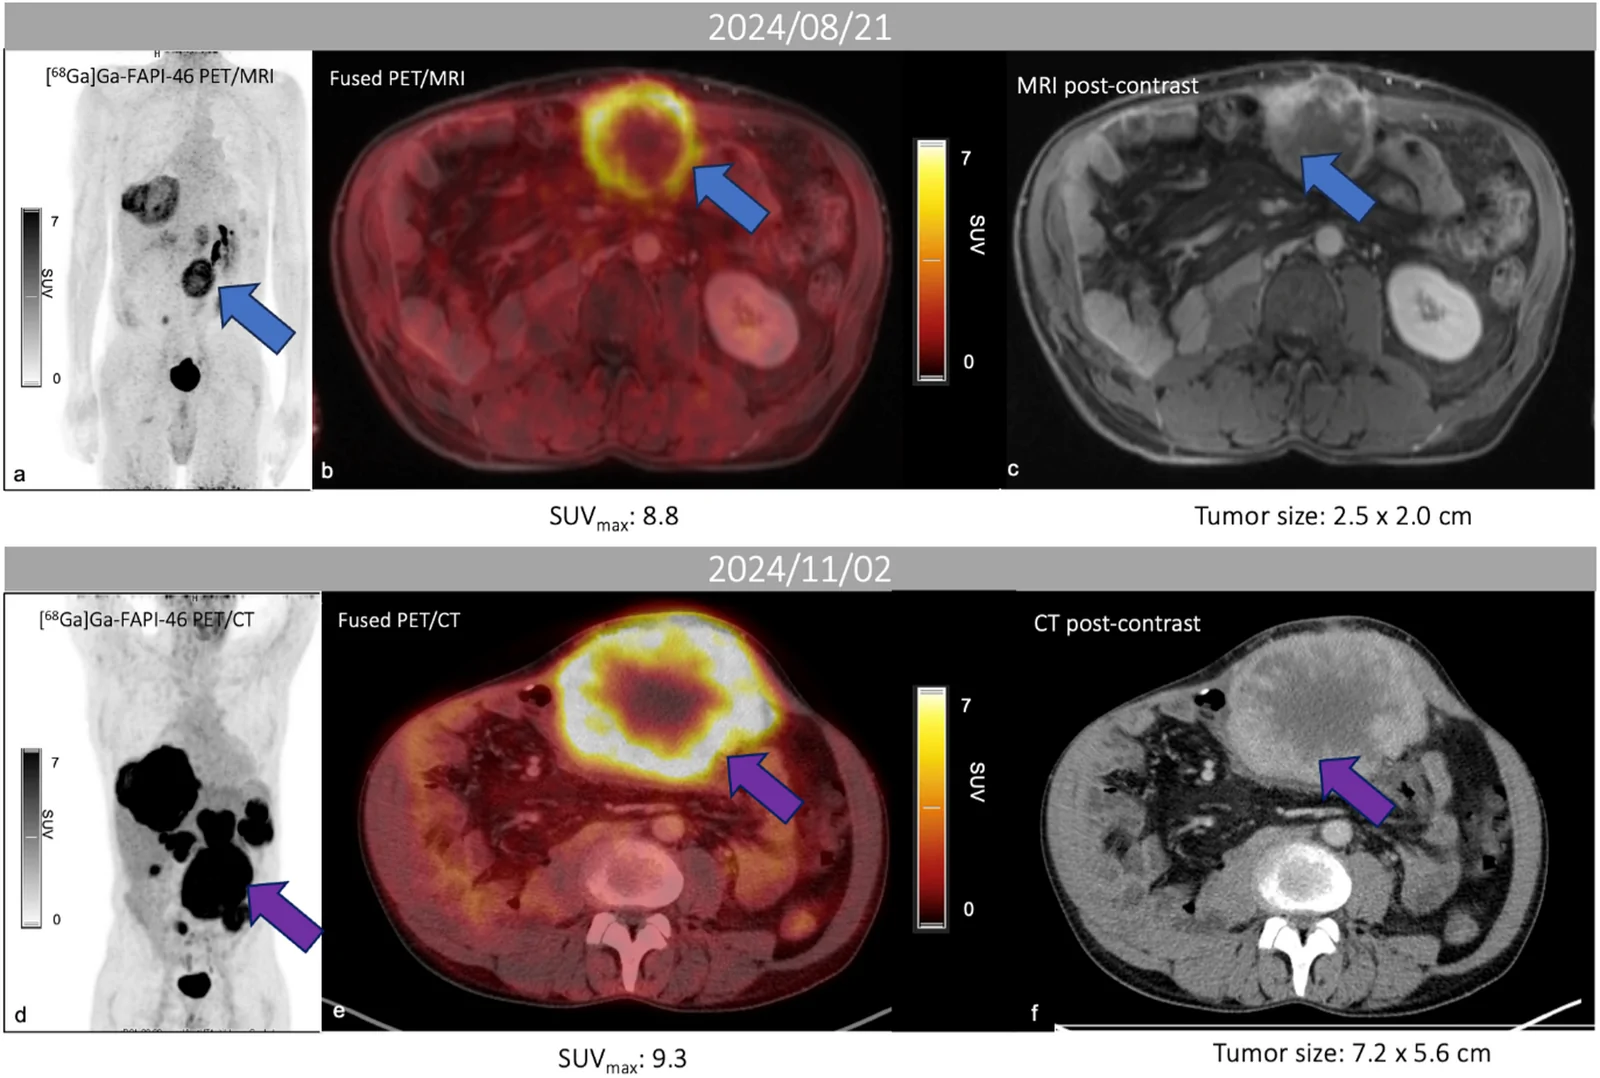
[^68^Ga]Ga‑FAPI‑46 PET/MRI before (a-c) and [^68^Ga]Ga‑FAPI‑46 PET/CT after (d-f) [^177^Lu]Lu-FAPI-46 treatment of a recurrent multifocal retroperitoneal DDLPS. The images demonstrate significant tumor progression under treatment, with significant increase in both lesion size and SUV_max_ with multiple newly delineated tumor lesions
